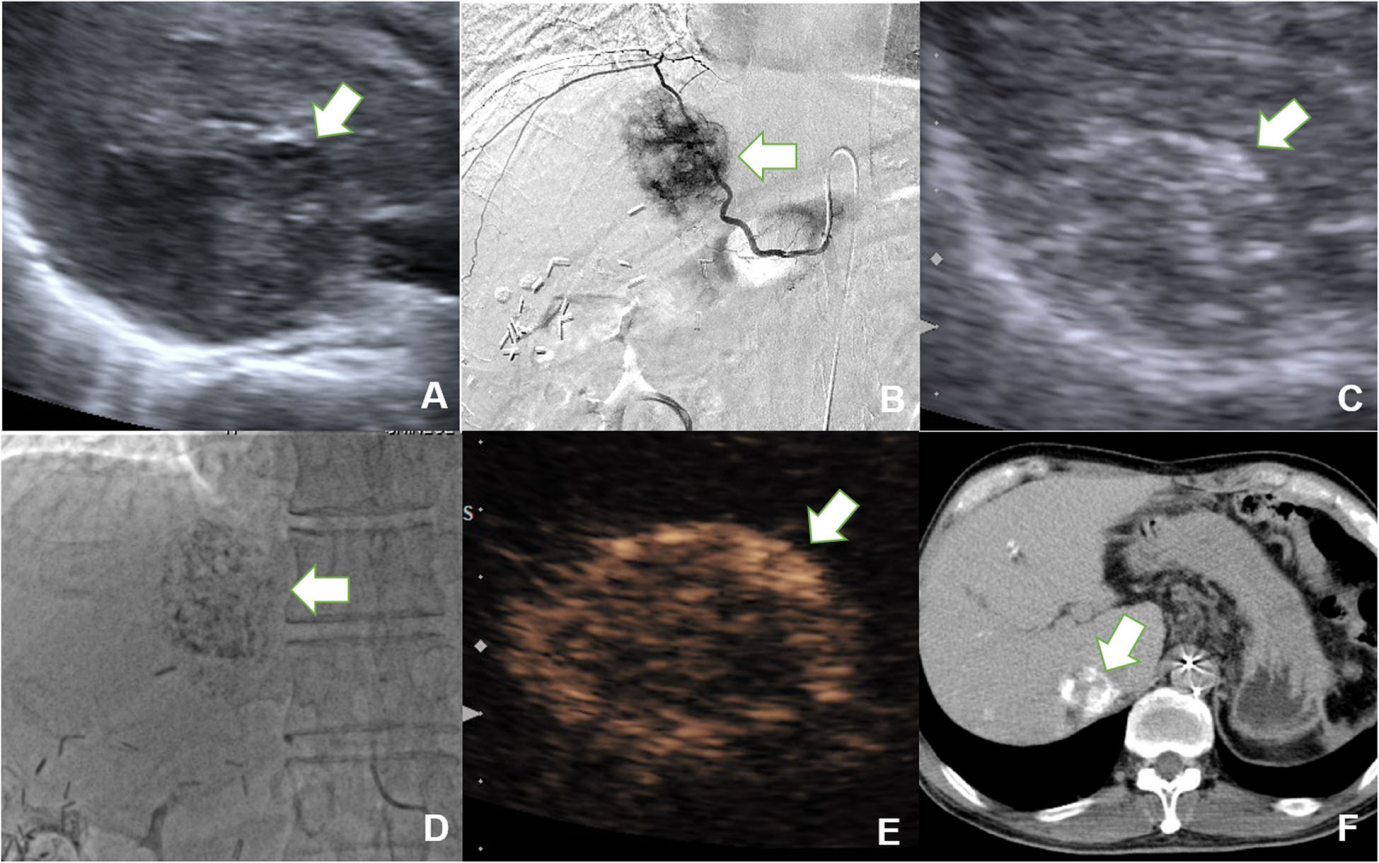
Comparison among HA-CUES, CBCT and DSA imaging. A, B-mode ultrasonography showed a hypoechoic HCC lesion located in segment 7 before TACE (arrow). B, Supperselected DSA showed the lesion before TACE (arrow). C, B-mode ultrasonography revealed lipiodol deposition in the lesion immediately after TACE (arrow). D, The lesion was shown on DSA after TACE (arrow). E, Postoperative HA-CEUS showed corona enhancement in the late phase (arrow). F, Postoperative cone-beam CT showed the high density deposition inside and around the tumour (arrow). HA-CEUS = hepatic artery contrast-enhanced ultrasound; DSA = digital subtraction angiography
